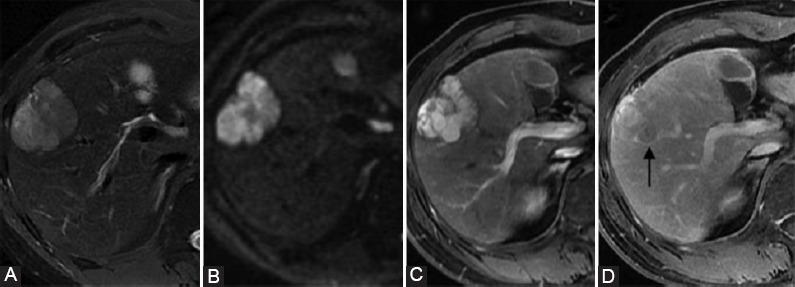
A surgically confirmed combined hepatocellular carcinoma-cholangiocarcinoma (cHCC-CCA) in a 43-year-old male with hepatitis B virus infection. (A) T2WI-FS image, (B) diffusion-weighted imaging (DWI) image (b = 1000 sec/mm), (C) late arterial, and (D) delayed phase images. The tumor showed high signal intensity on T2WI-FS and DWI images arterial phase hyperenhancement (not rim) during the late arterial phase and washout (not peripheral) during the delayed phase. (D) In delayed phase, the tumor showed lobulated capsule appearance (arrow).
